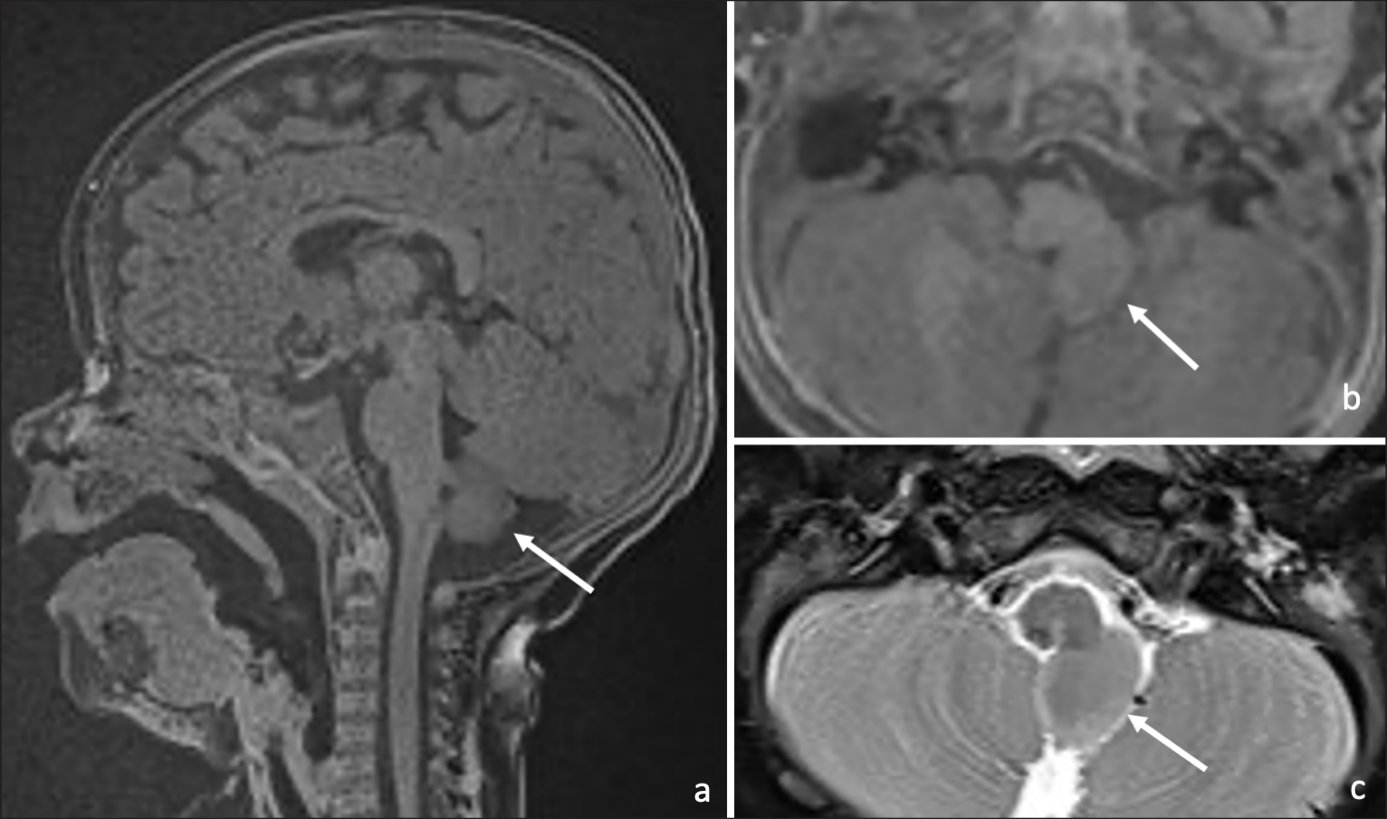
On both T1- and T2-weighted images, the signal intensity of the lesion was identical to that of the adjacent cerebellum, with white matter signal intensity in the center and cortical signal intensity in the periphery of the lesion. Mainly on the T1-weighted imaging, discrete foliation was noted. The lesion showed no diffusion restriction or enhancement after IV gadolinium administration (Figure 2: [a] sagittal 3D fat-suppressed T1-weighted image showing the central white matter intensity (dashed arrow) and peripheral cerebellar foliation (arrow) of the seminodular mass, also note the presence of a dysplastic superior soft tissue bridge (arrowhead); [b] axial fat-suppressed T2-weighted spin echo image showing a normal medulla oblongata on the right side (arrow)).
